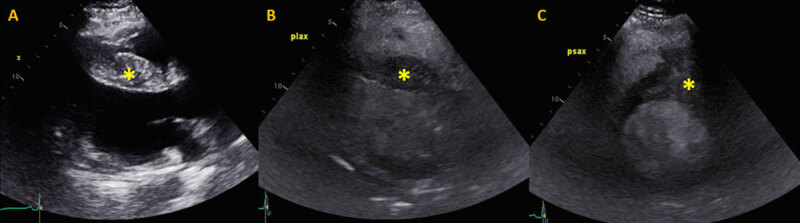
Preoperative transthoracic echocardiogram. (A) Transthoracic echocardiogram without contrast and (B, C) with intravenous contrast-enhancing agent. (B) Parasternal long axis view demonstrating asymmetric septal thickening/mass with contrast enhancement and (C) protrusion of the mass into the right ventricular outflow tract on parasternal short axis.
